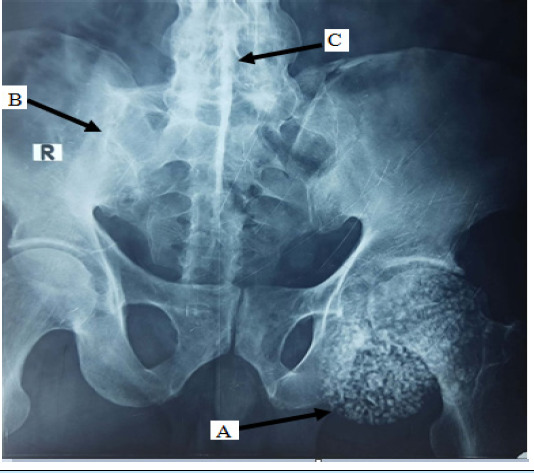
X-ray showing A) Preoperative radiograph of the right hip joint with features of synovial chondromatosis, B) Fused right-sided sacroiliac joint, and C) 'Dagger' signs which are suggestive of ankylosing spondylitis.
